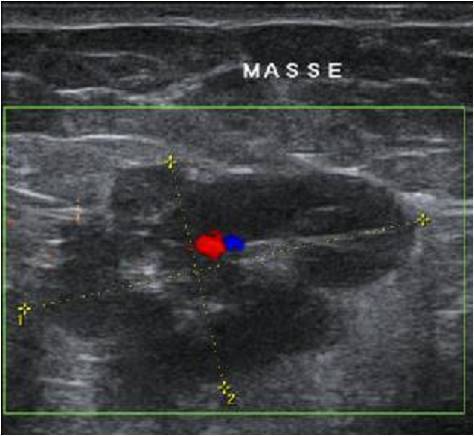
L'échographique montrant une image hypoéchogène hétérogène à contours irréguliers, et vascularisée au doppler couleur
